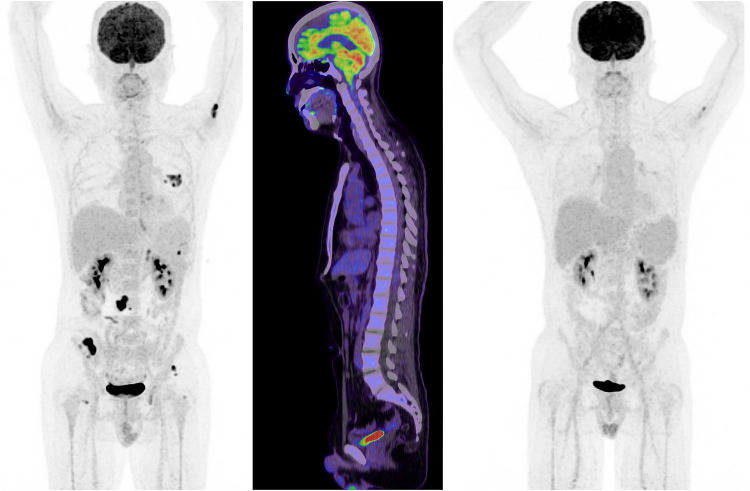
18F-FDG PET/CT maximum intensity projection (MIP) images and sagittal fused PET/CT images. Staging 18F-FDG PET/CT maximum intensity projection (MIP) image (left side) showing FDG-avid left lung, adrenal lesion and some bone metastases (left humerus, right iliac wing). Sagittal fused PET/CT image (middle image) showing no evidence of meningeal involvement. Follow-up PET/CT MIP image (right side) demonstrates near complete resolution of lung, adrenal and right iliac wing lesion as well as decreasing uptake in the left humerus.
